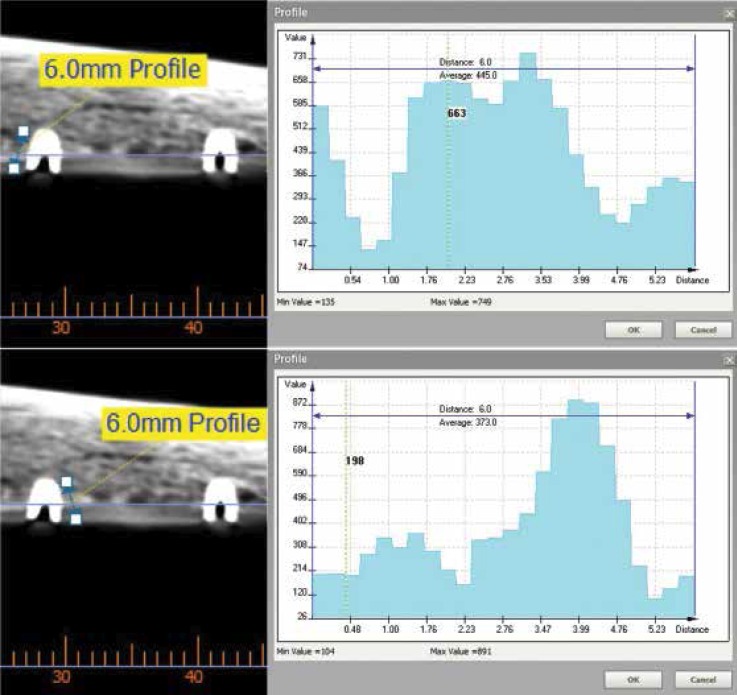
Cross-sectional images of a CT scan performed in one of the ribs using Ez3D Plus software.
